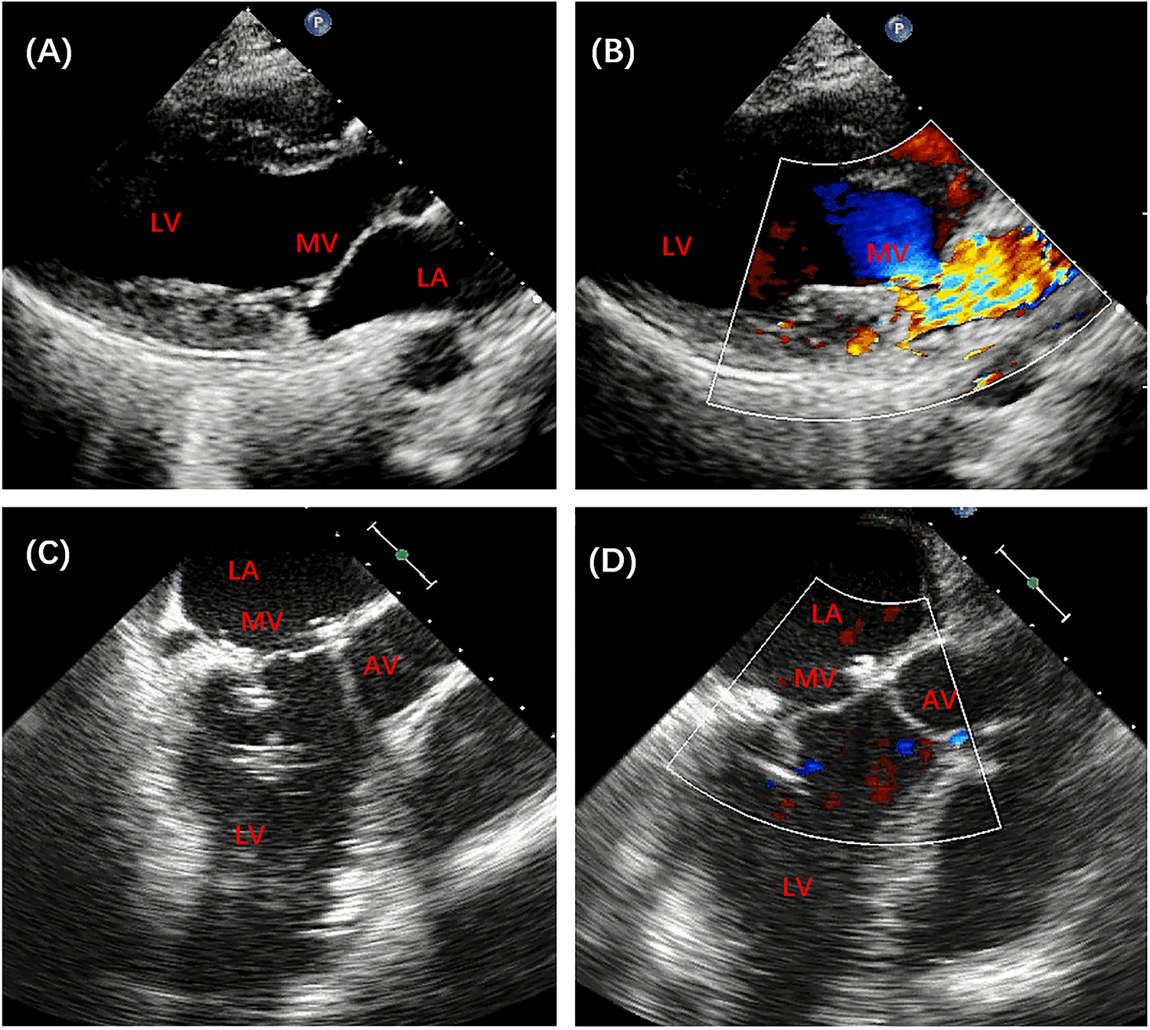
Preoperative and postoperative echocardiography of patient no. 13. (A,B) Preoperative echocardiography revealed severe mitral insufficiency. (C,D) Postoperative echocardiography showed good mitral valve function. AV, aortic valve; MV, mitral valve; LA, left atrium; LV, left ventricle.
